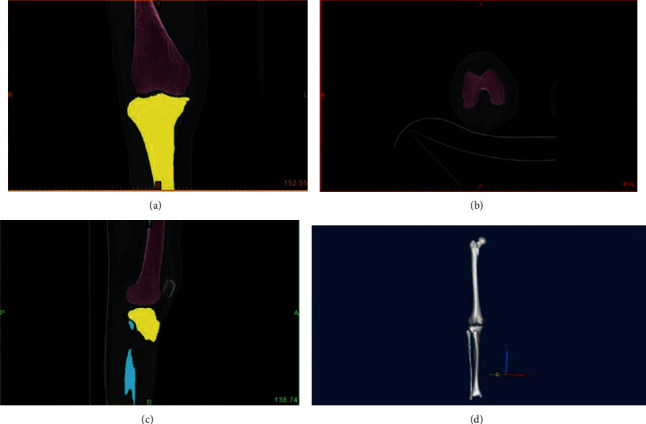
(a) Front view CT image of the knee joint. (b) Top view CT image of the knee joint. (c) Side view CT image of the knee joint. (d) Reconstructed three-dimensional model of a lower limb in full length.
